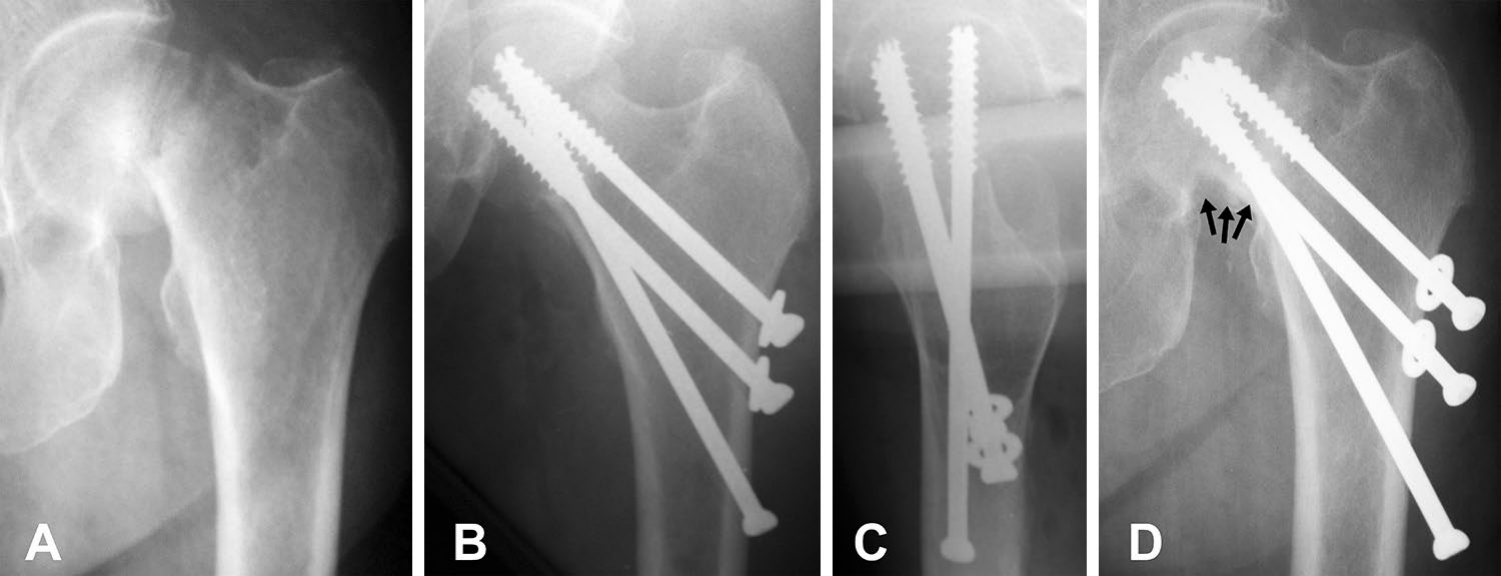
Exemplified X-rays of a 73 yo female patient with incomplete fixation failure and fragment impaction as a result of extreme loading after BDSF treatment: AP view diagnostics (a), postoperative AP view (b), postoperative lateral view (c), AP view 20-day follow-up with incomplete fixation failure and excessive fragment impaction, the latter denoted by *black arrows* (d). This patient died of acute renal insufficiency. Although severe traumatic agent caused partial displacement, the proper positioning of the screws prevents total displacement and transforms the shearing forces into compressive, causing further impaction
